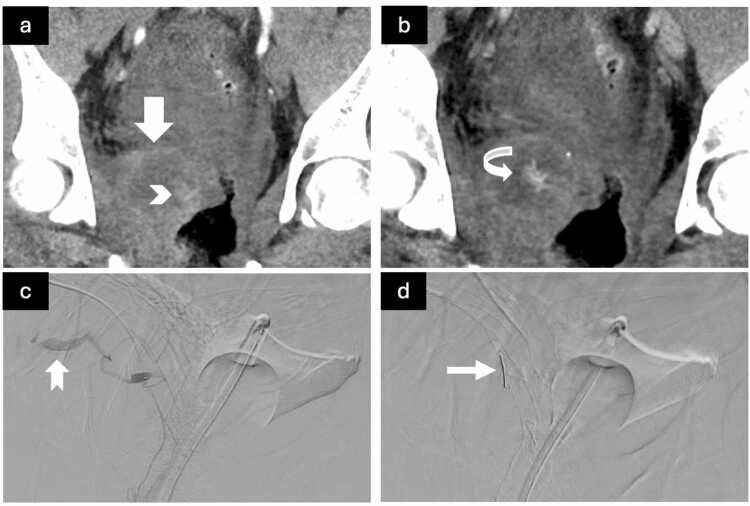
30year – old female with intrapartum 2nd degree vaginal tear repaired in OR. 8 h postpartum patient dropped hemoglobin. Coronal CT pelvis (a) arterial phase (b) delayed phase shows a large pelvic hematoma along the right cervicovaginal wall (bold arrow) with faint contrast extravasation on arterial phase (chevron) and contrast pooling on the delayed phase (curved arrow). Digital subtraction angiography (c) confirms active hemorrhage from vaginal branch of the right uterine artery (notched arrow) (d) successfully embolized (thin arrow). DIAGNOSIS: postpartum haemorrhage.
